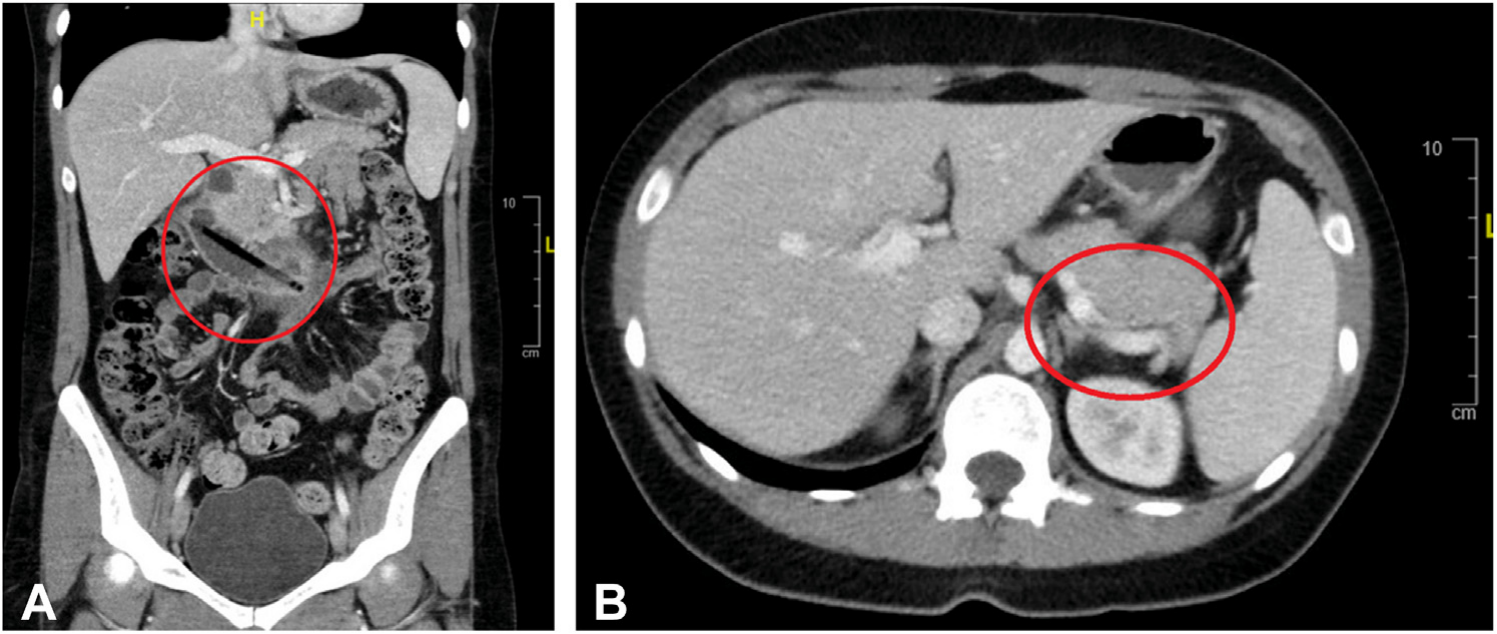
CT scan anteroposterior with intravenous contrast showed coronal section (A) and horizontal section (B) ingested “pen-like” 11- × 0.5-cm foreign body at the second and third portion of the duodenum. The foreign body deep laceration 2 cm through the second portion of the duodenum at the right lateral wall, adjacent to trace subhepatic and small pelvic free fluid. Additional duodenal wall thickening/inflammation at the third portion without additional deep laceration.
